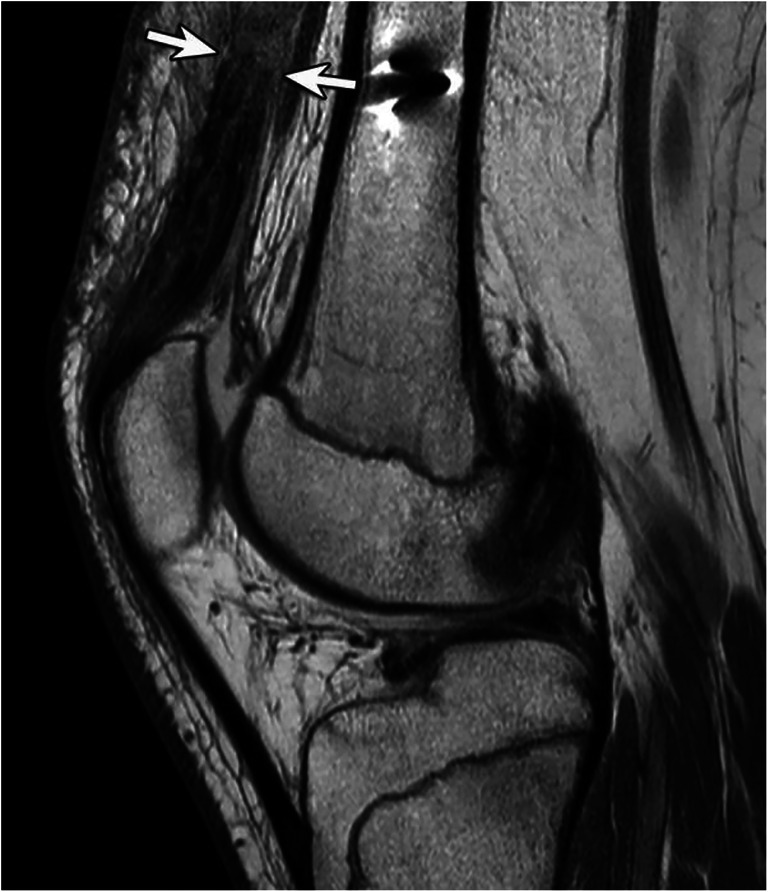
Sixteen-year-old male with quadriceps femoris tear. Intermediate-weighted MR image without fat saturation shows a complete quadriceps tendon rupture at the myotendinous junction (arrows). The patient was treated surgically with an end-to-end tendon repair
